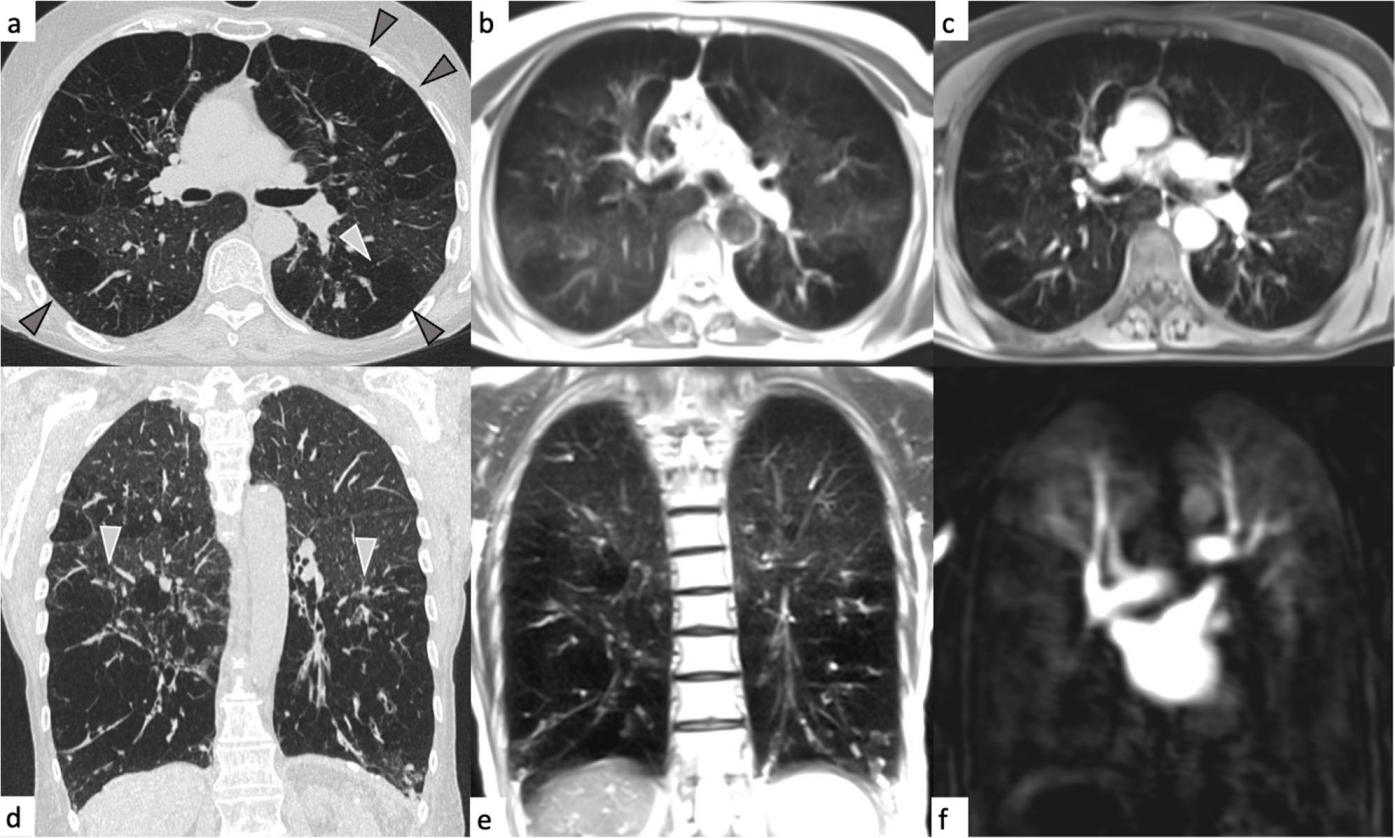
Mixed pattern with emphysema and extensive airway disease in a 62-year-old female participant (classified as airway predominant COPD phenotype). Arrowheads on the CT images (a, d) indicate severe emphysema in both anterior upper and posterior lung lobes. On the corresponding MRI, the affected parts of the lung appear as signal void in the transverse half Fourier fast spin echo images (b, e) or lung parenchyma defects on the contrast-enhanced transverse 3D gradient echo image (c) and as extensive perfusion deficits in the DCE series (f)
